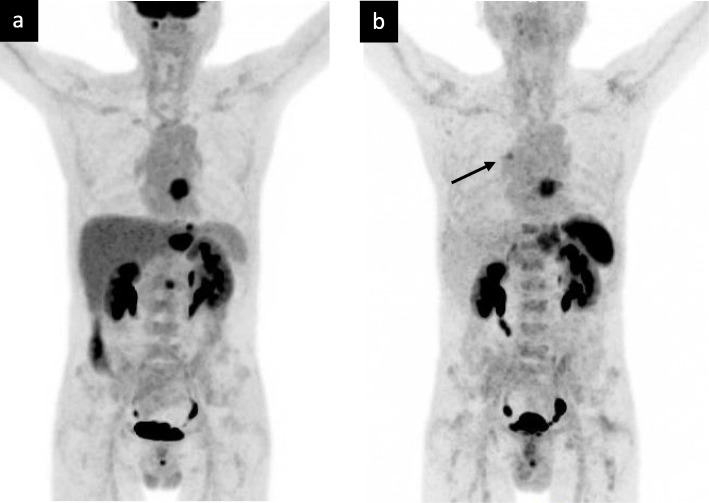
Key lesion with close relation to the primary tumor: Esophageal carcinoma patient (#4) with (a) negative FDG-uptake and an additional suspicious lesion hilary right enhanced by Pentixafor PET/CT (b). The abdominal uptake is caused by an inserted gastric feeding tube. Arrow indicates the hilary lesion
